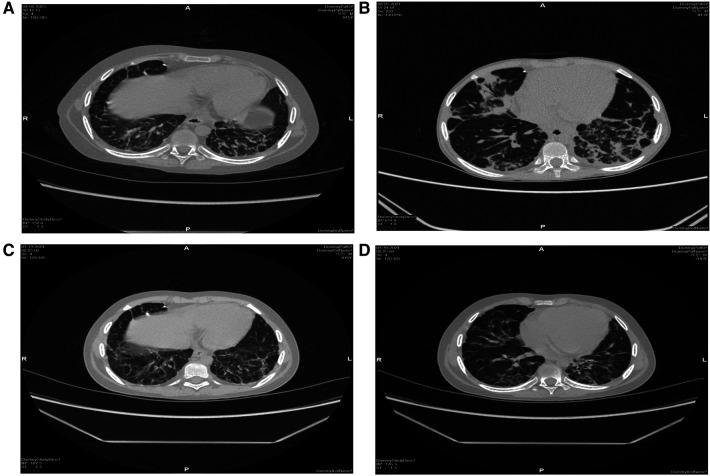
High-resolution CT scan of the lungs. Before transplantation, bilateral pulmonary fibrosis with segmental consolidation in the left lower lobe (A,B), consolidation was significantly absorbed, and fibrosis improved after transplantation (C,D).
